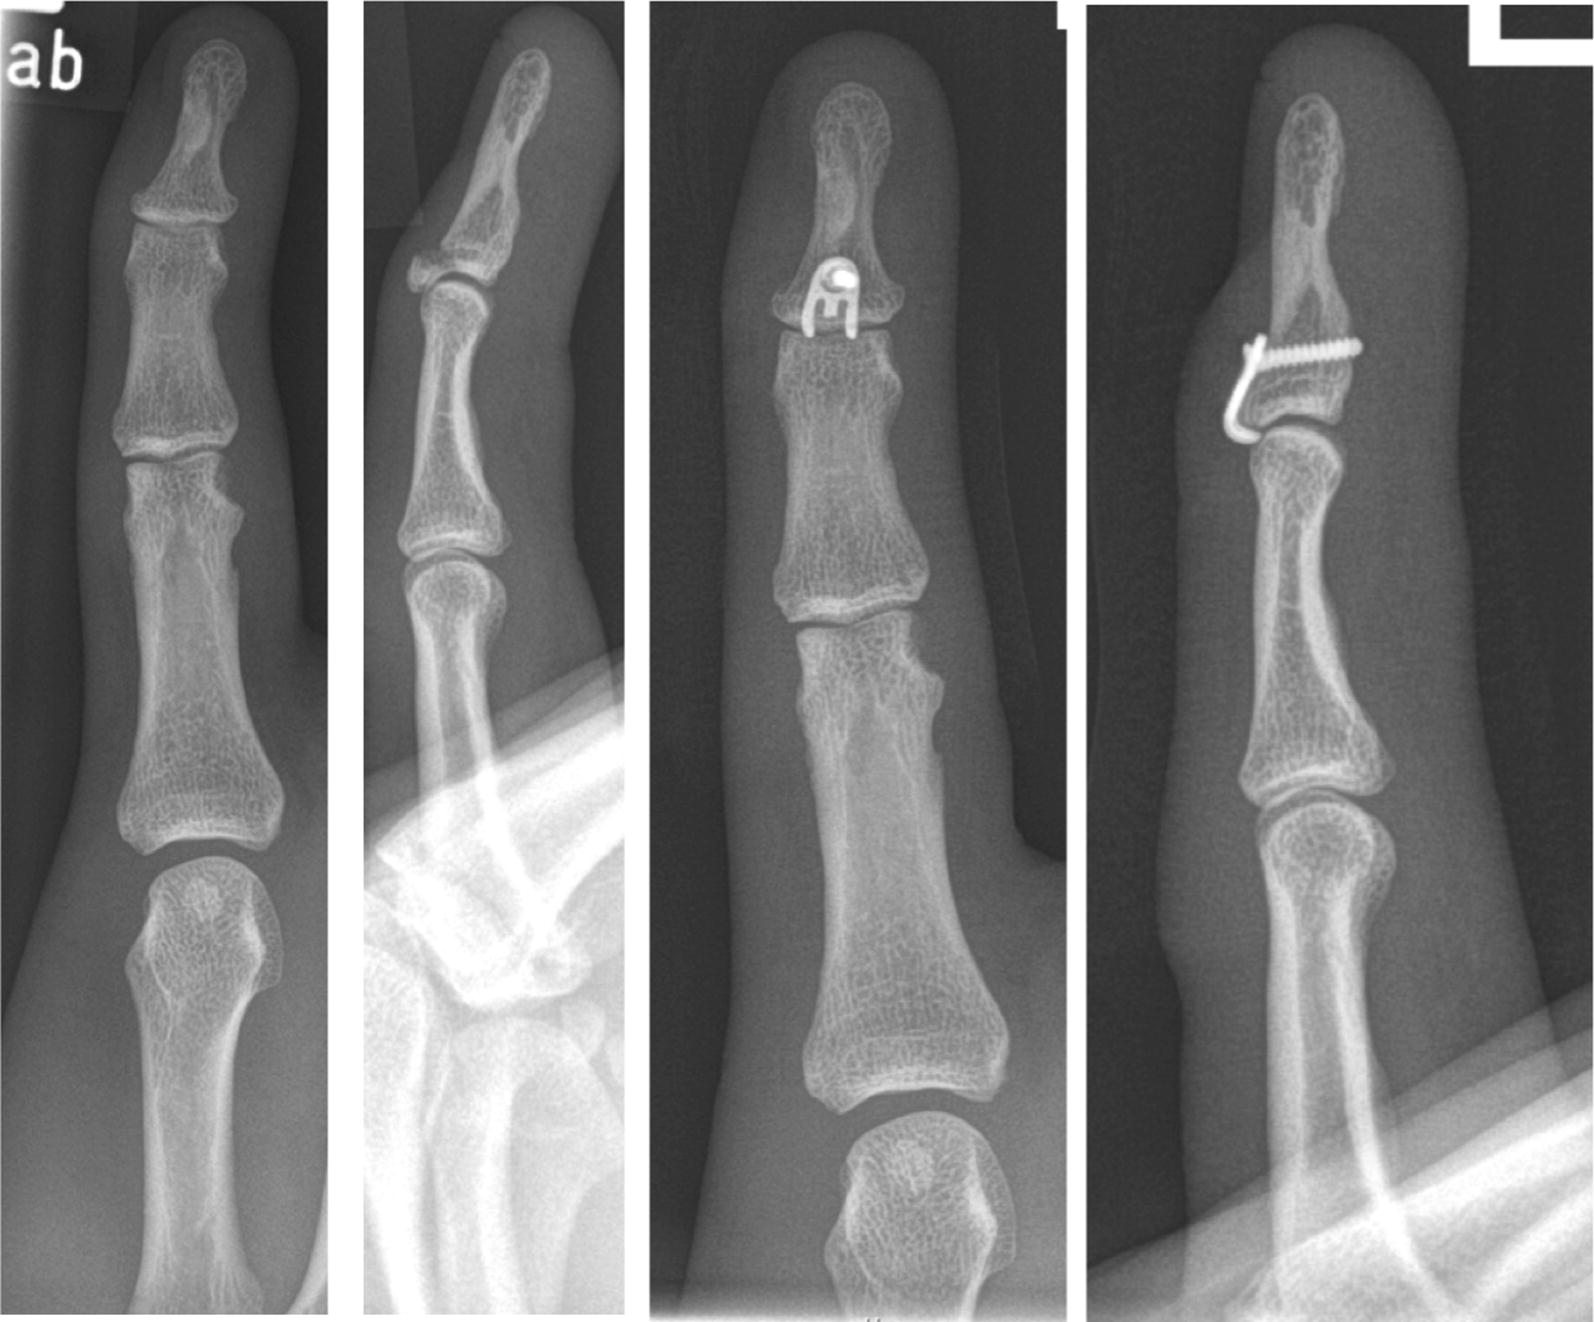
A distal phalanx fracture with dislocation and the postoperative x-ray after treatment with a hook plate
